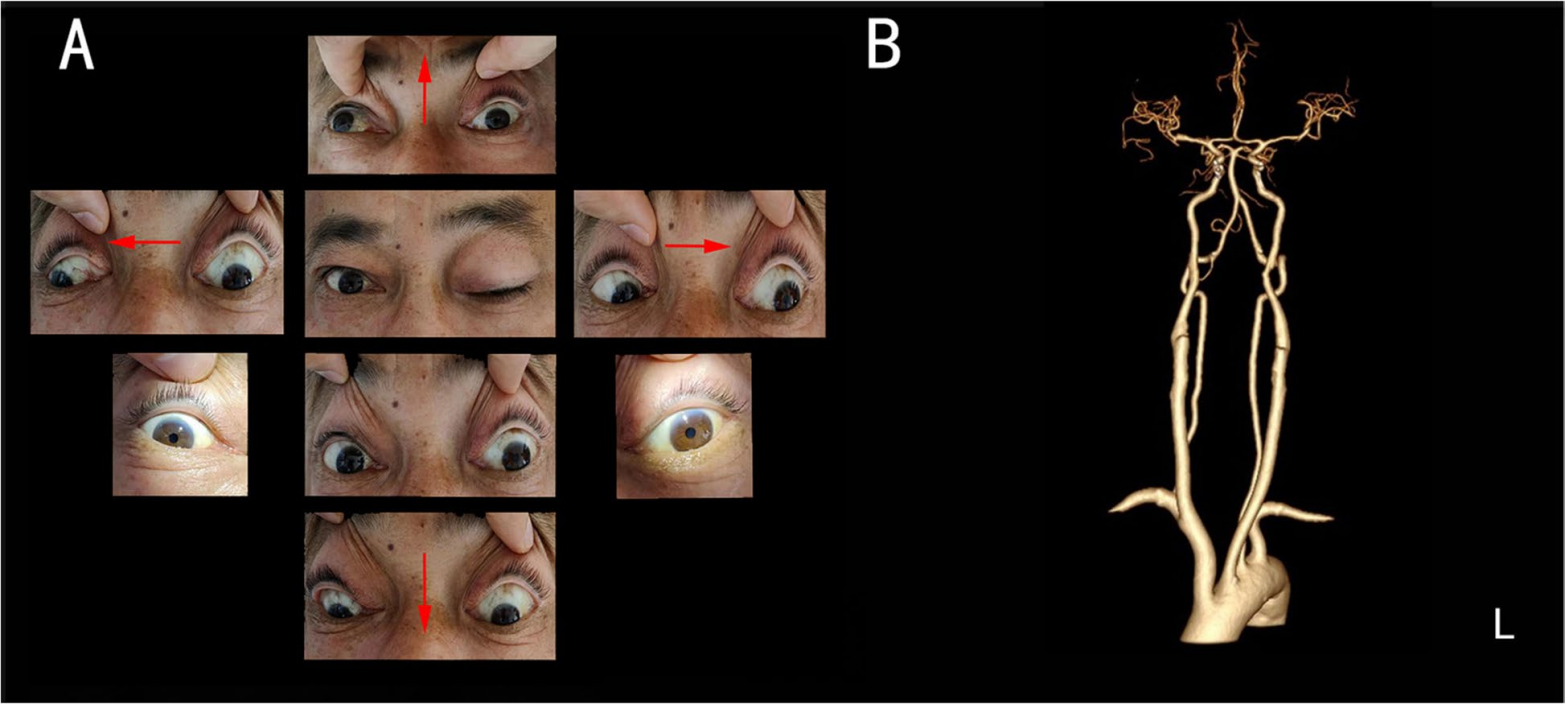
The pupillary sphincter is selectively spared in recorded eye movements (A); CT angiography of the brain vessels suggest the left vertebral artery originated directly from aortic arch (B). Abbreviations: L, left
